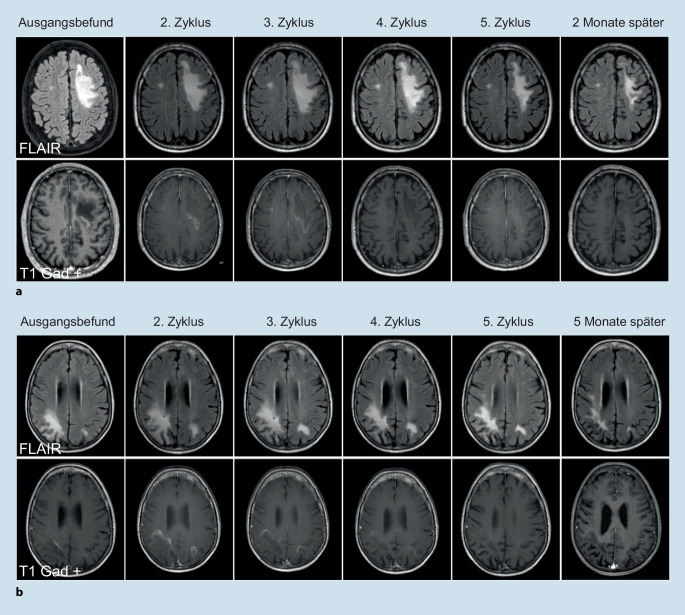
Nach Übernahme in unsere Klinik begannen wir eine Therapie mit dem Immuncheckpointinhibitor Pembrolizumab (2 mg/kg Körpergewicht) in 4‑wöchentlichen Abständen. Zu Beginn des 3. Zyklus kam es zu einer leichten Zunahme der Aphasie und korrelierenden Größenprogredienz der Läsion mit weiterhin randständiger Kontrastmittelanreicherung in der MRT-Bildgebung (Abb. 1a). Nach 2 weiteren Zyklen verbesserte sich jedoch die Armparese. Die FLAIR-hyperintensen Marklagerveränderungen und die randständige Kontrastmittelanreicherung waren regredient. Passend dazu war die Kopienzahl der JC-Virus-DNA im Liquor rückläufig und zu Beginn des 4. Zyklus nicht mehr nachweisbar.
